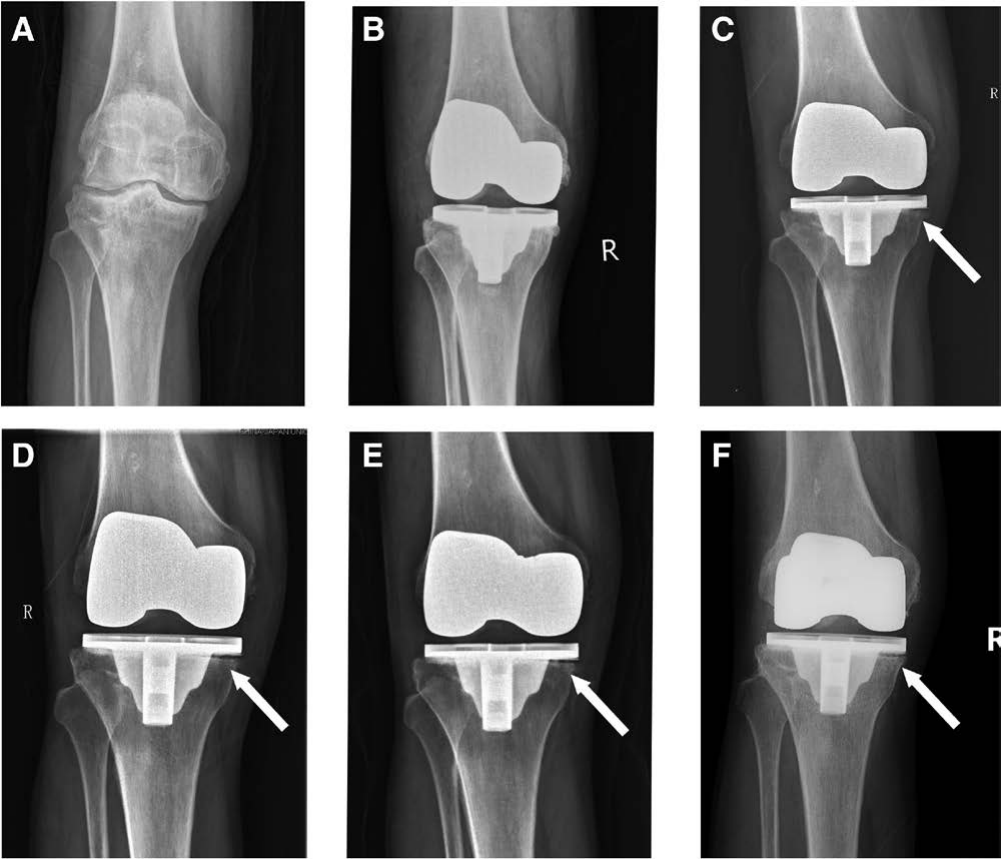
(A–F) AP radiographic progression of the right knee: (A) Preoperative AP radiograph demonstrating severe osteoarthritis; (B) postoperative radiograph following primary total knee arthroplasty; (C) AP radiograph taken 2 mo after DAIR, showing a radiolucent line beneath the medial tibial tray; (D) at the 5-mo follow-up, the radiolucent line has progressed; (E) at the 1-yr follow-up, the radiolucent line remains unchanged; (F) at the 5-yr follow-up, new bone formation is observed below the tibial component. AP = anteroposterior, DAIR = debridement, antibiotics, and implant retention.
